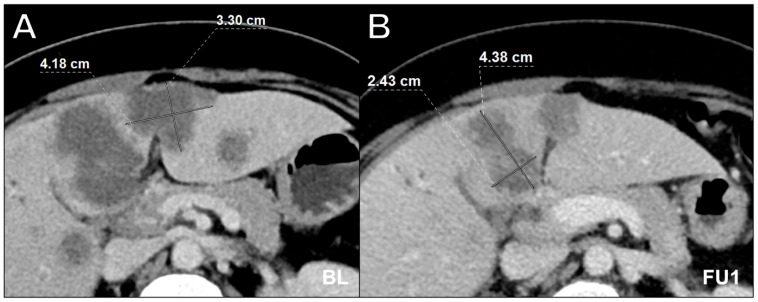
Axial contrast-enhanced computed tomography scan in portal venous phase in a 64-year-old male with multiple hepatic metastases in colorectal carcinoma at baseline (A) and follow-up 1 (B) showing incorrect lesion assessment at follow-up 1. Appropriate documentation is essential, especially when there are multiple target lesion candidates. While the software-assisted approach offers side-by-side assessment with structured lesion documentation, reader 3, who performed the manual assessment, mixed up lesions at follow-up 1, resulting in an incorrect overall response compared to the consensus reading. It is likely that the presentation state was not correctly stored in the PACS when the baseline measurement of the lesions was performed. BL = baseline, FU1 = follow-up 1.
